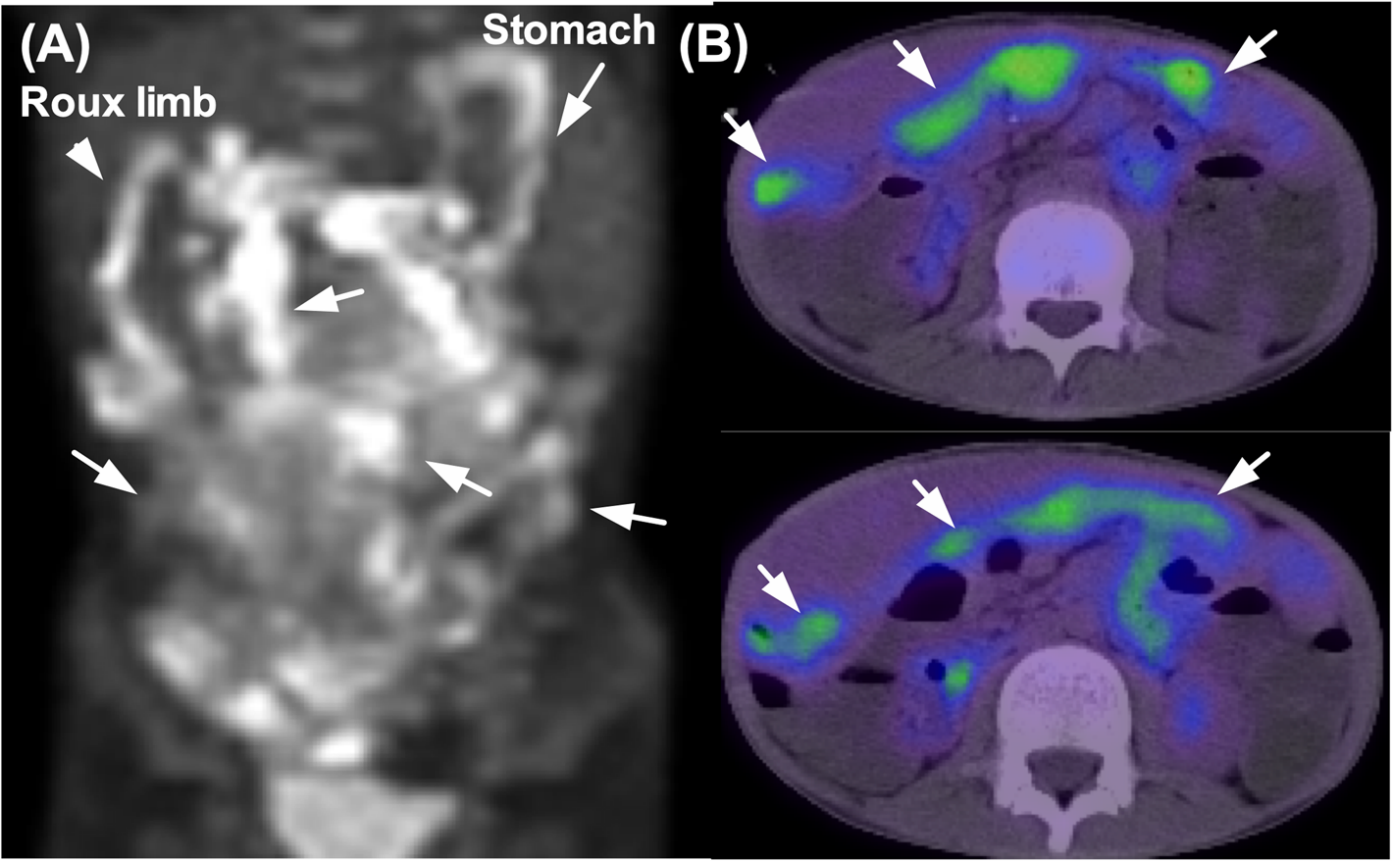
PET-CT: PET-CT revealed a wide range of the small intestine increased FDG activity. a The image of the whole abdomen in FDG activity. a, b The arrowhead indicates the jejunum of the Roux limb. The arrows indicate the stomach and small intestine with increased FDG activity
